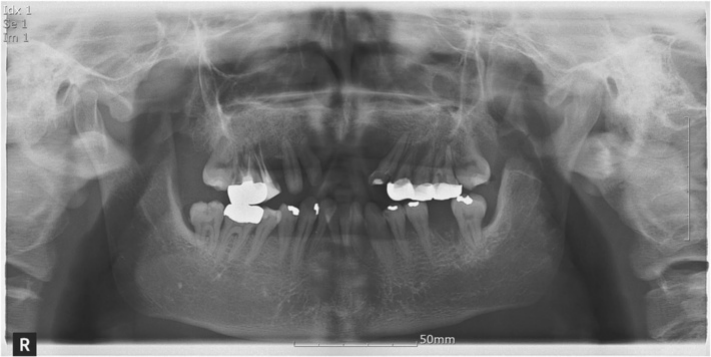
Panoramic radiography shows the fracture of the left mandibular coronoid process
